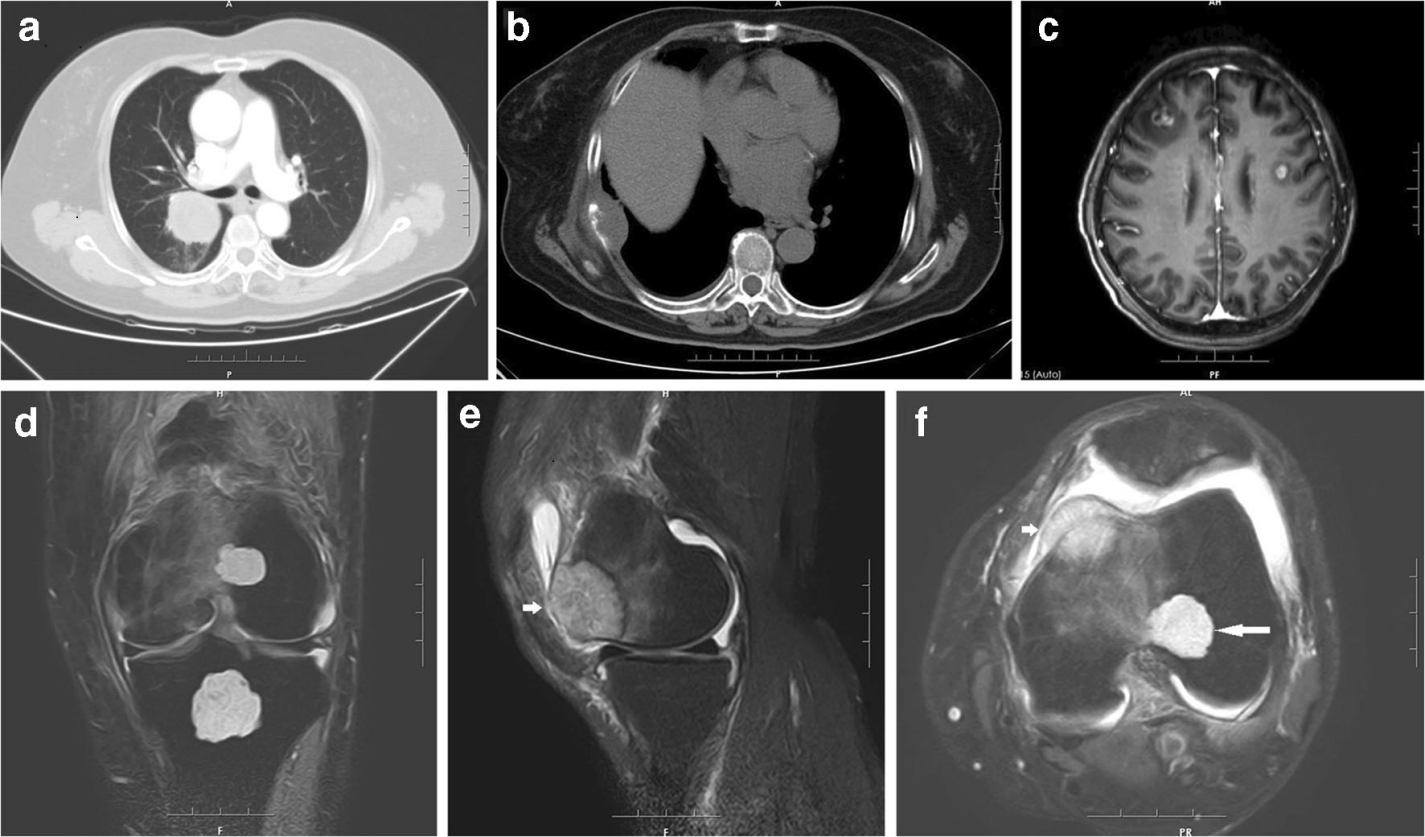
a Contrast-enhanced CT scan of the chest revealed a mass with irregular margin in the posterior segment of the right upper lobe of the lung. b Osteolytic metastasis in the right 7th rib with soft tissue mass formation. c Contrast-enhanced MRI image of the brain showed multiple metastases in the bilateral frontal lobes. d–f Coronal (d), sagittal (e) and axial (f) fat-suppressed T2-weighted MRI images demonstrated three lesions in the left femur and tibia, including a lesion in the tibia, a lesion in the anterior part of the medial condyle of femur (short arrow, metastatic carcinoma) and a lesion located in the lateral condyle (long arrow, co-existing tumour of metastasis and enchondroma)
